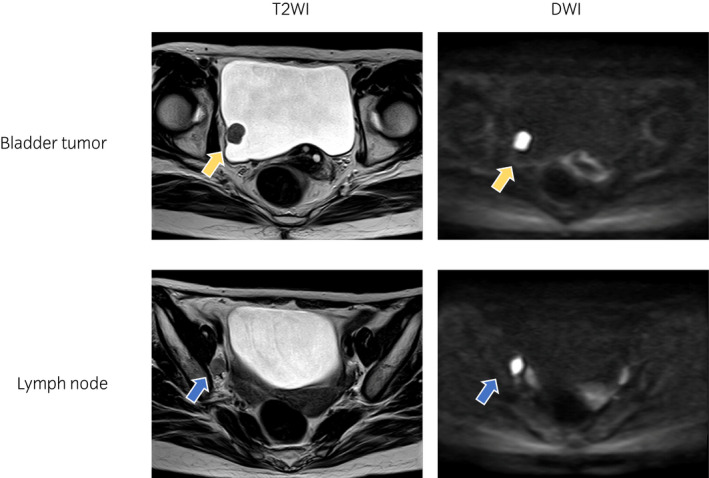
MRI of the bladder showed a solid tumor without muscle invasion (yellow arrow) and right obturator lymph node swelling (blue arrow). [Colour figure can be viewed at wileyonlinelibrary.com]
